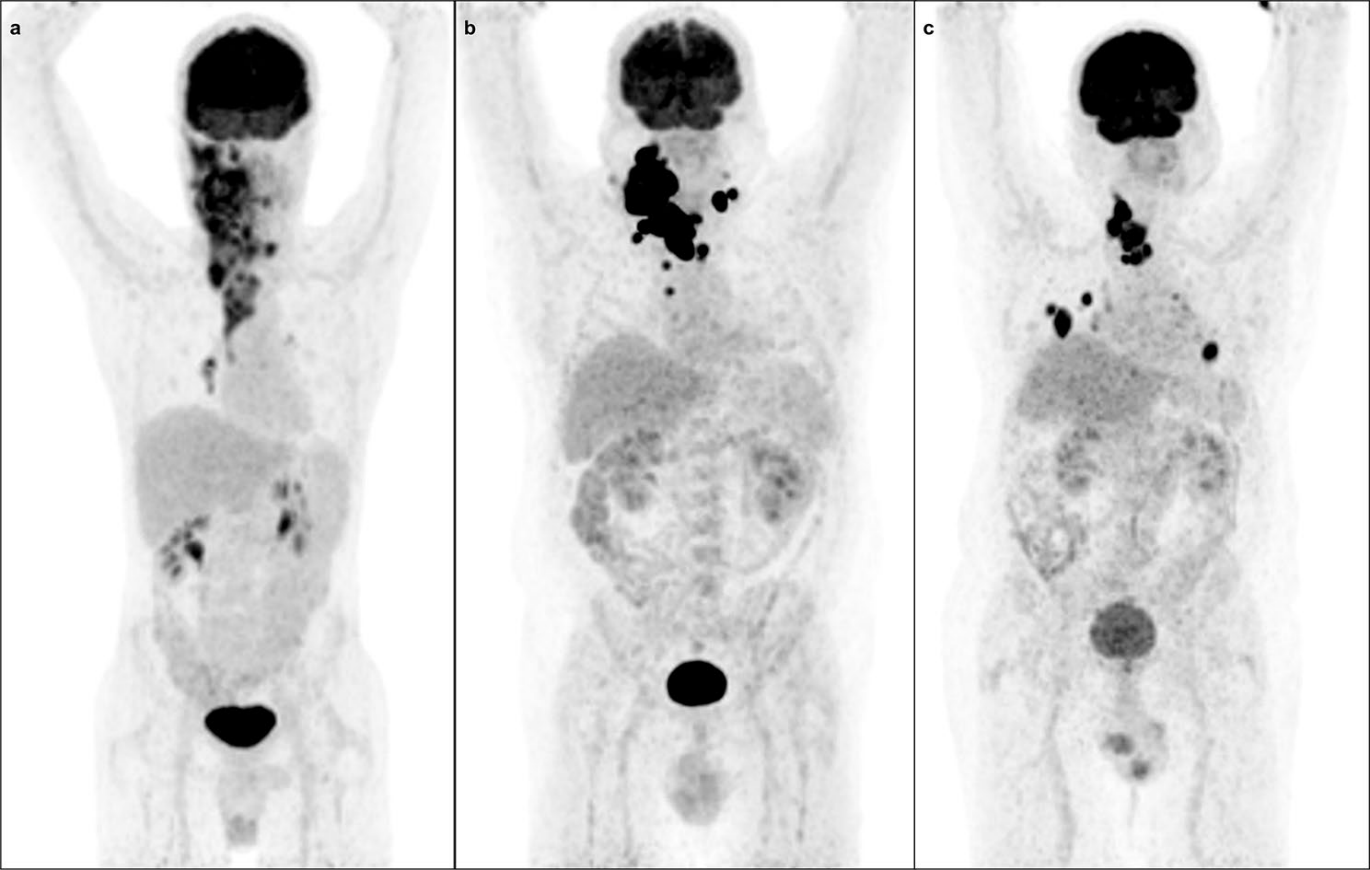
[^18^F]FDG PET/CT performed during initial evaluation. (a) A 34-year-old man with anaplastic thyroid carcinoma with metastases to the lymph nodes in the right lung hilum. (b) A 56-year-old man with squamous cell carcinoma of the thyroid (classified as anaplastic thyroid carcinoma according to the new WHO classification) with metastases to the mediastinal lymph nodes. (c) A 74-year-old man with anaplastic thyroid carcinoma with metastases to the lungs, mediastinal lymph nodes and lung hilum
